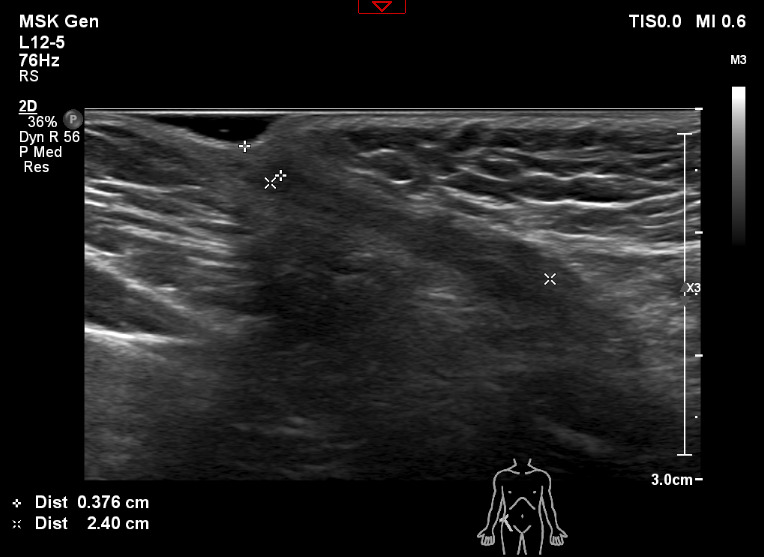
Longitudinal ultrasound image of the foreign body identified in the inguinal region.
